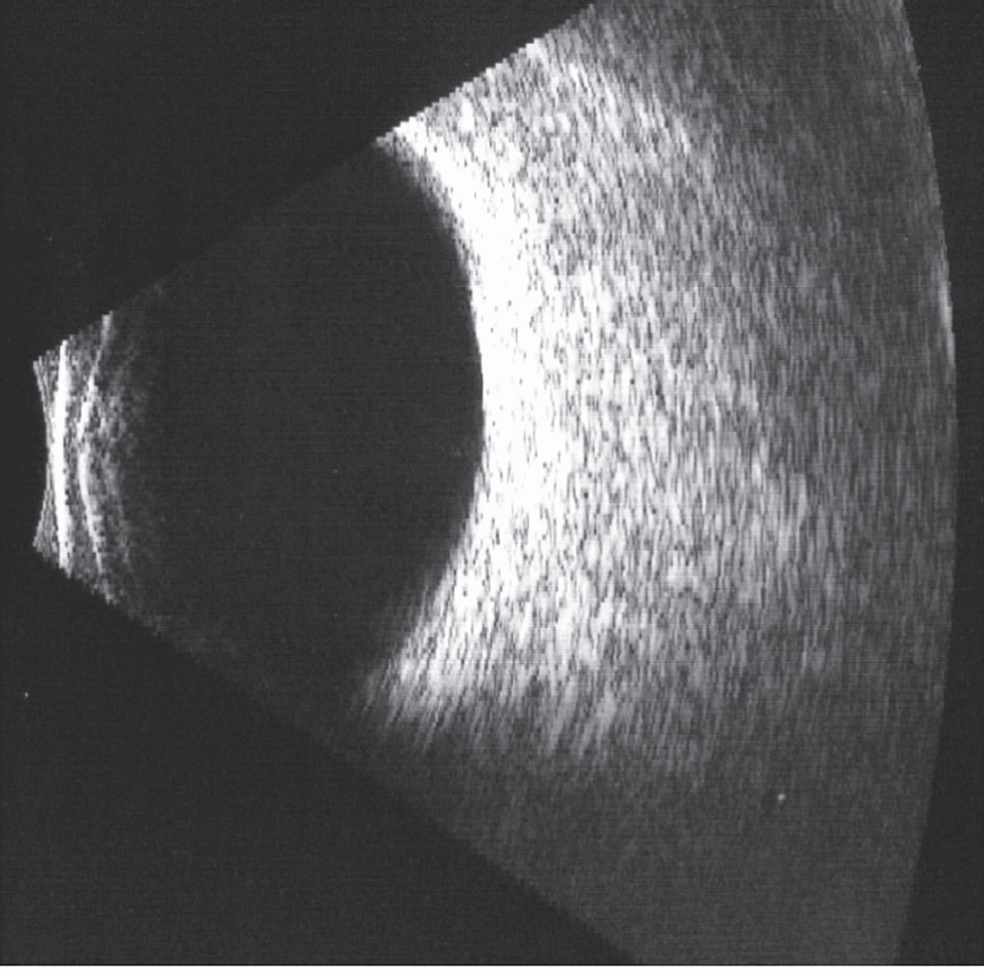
Bright scan ultrasonography of the right eye.
